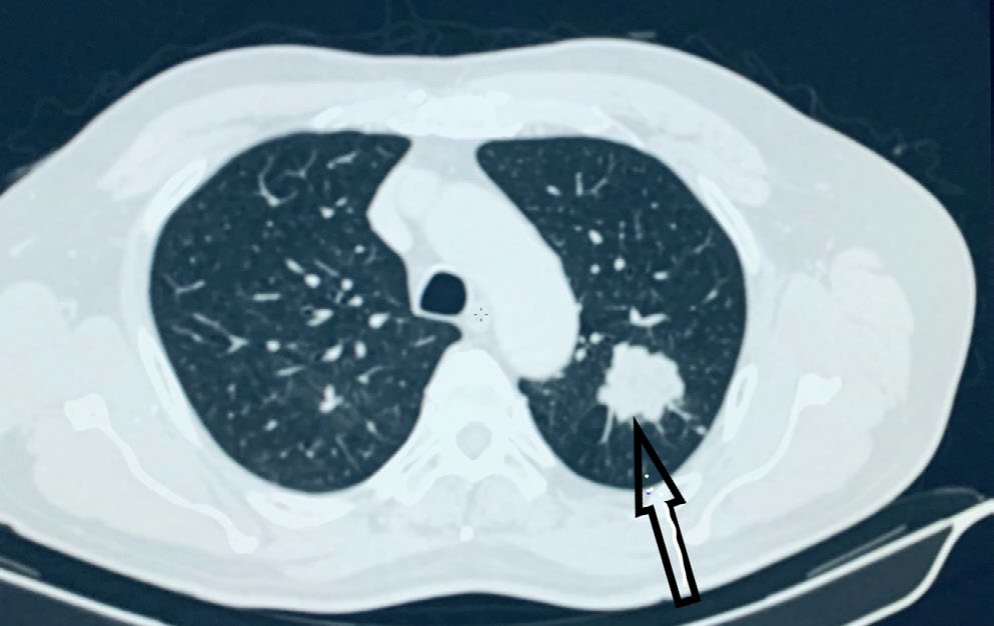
CT Scan of the chest shows a minimally enhancing lesion measuring 3.3 × 3.2 cm with lobulated margins(as indicated by an arrow)
